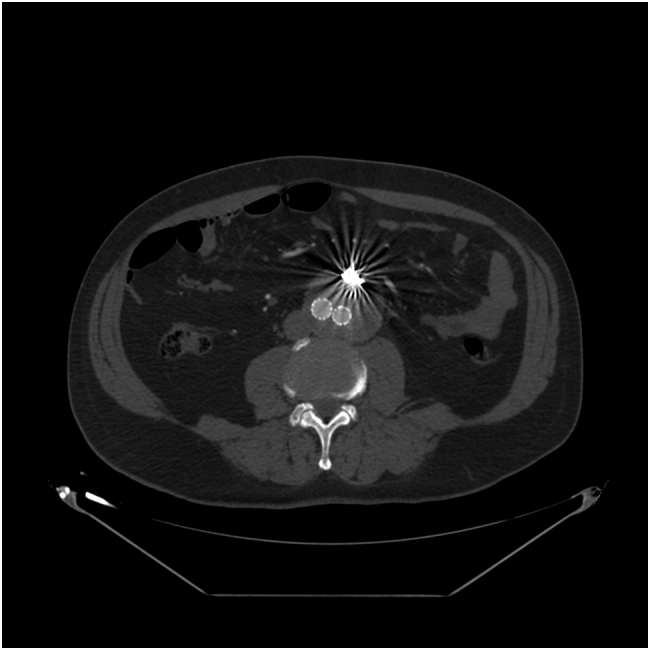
CT-angiography at 6 months after embolization showing complete type 2 endoleak sealing.
